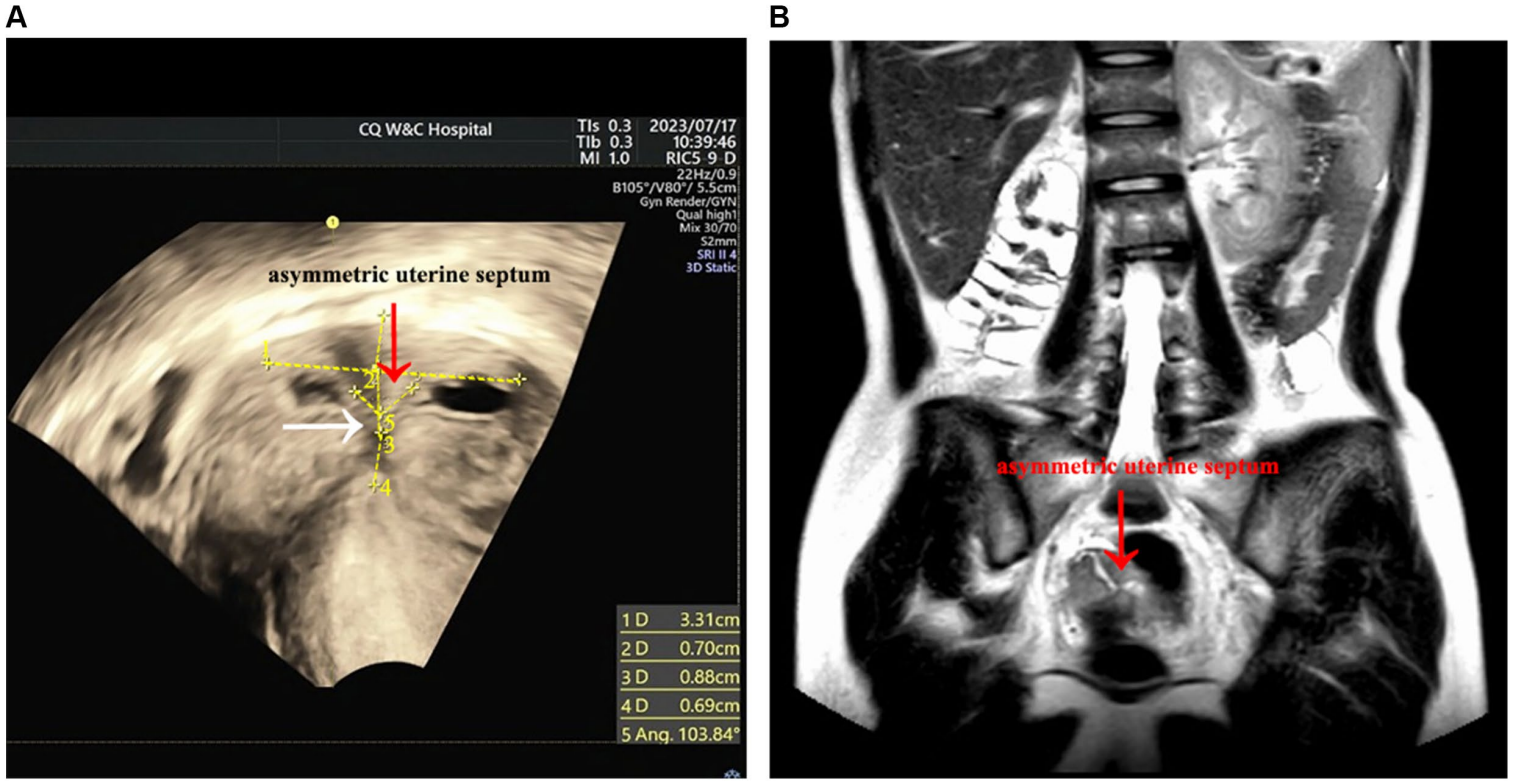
Preoperative imaging examination. (A) The red arrow points to the asymmetrical uterine septum, while the white arrow points to the obstruction site, i.e., the transverse uterine septum. However, the ultrasound was not completely clear, and the transverse uterine septum was not visualized. (B) The uterine fundus, indicated by the arrow, is depressed downward toward the uterine septum. Given that the thickness of the transverse uterine septum was below the MRI slice gap, the MRI image did not capture the transverse uterine septum.
